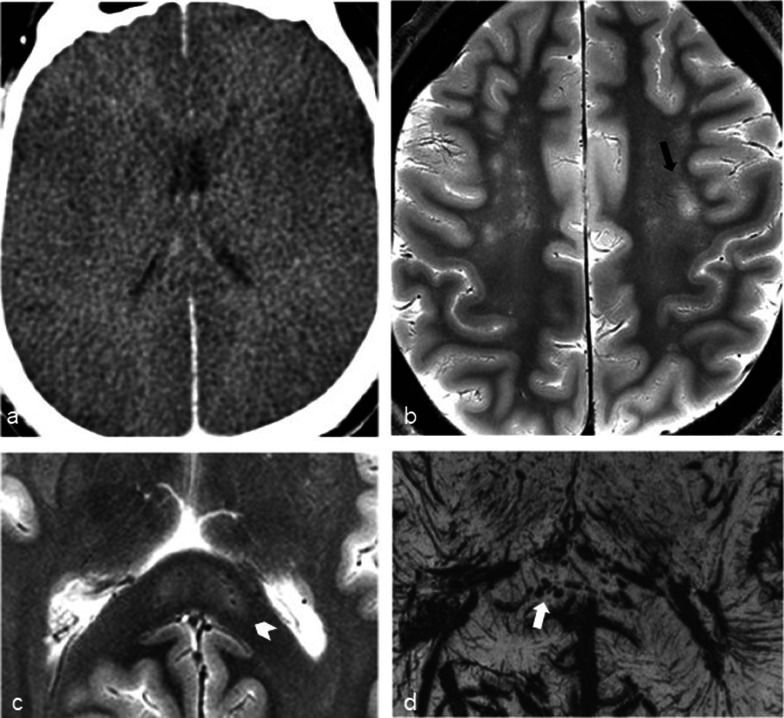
Diffuse edema (CT a) and some patchy white matter focal abnormalities (axial T2 b) (black arrow) in patient 3. Patient 4 had splenial signal abnormality (white arrowhead) with high T2 signal (c) with punctate hemorrhagic foci on SWI (d) (white arrow)
